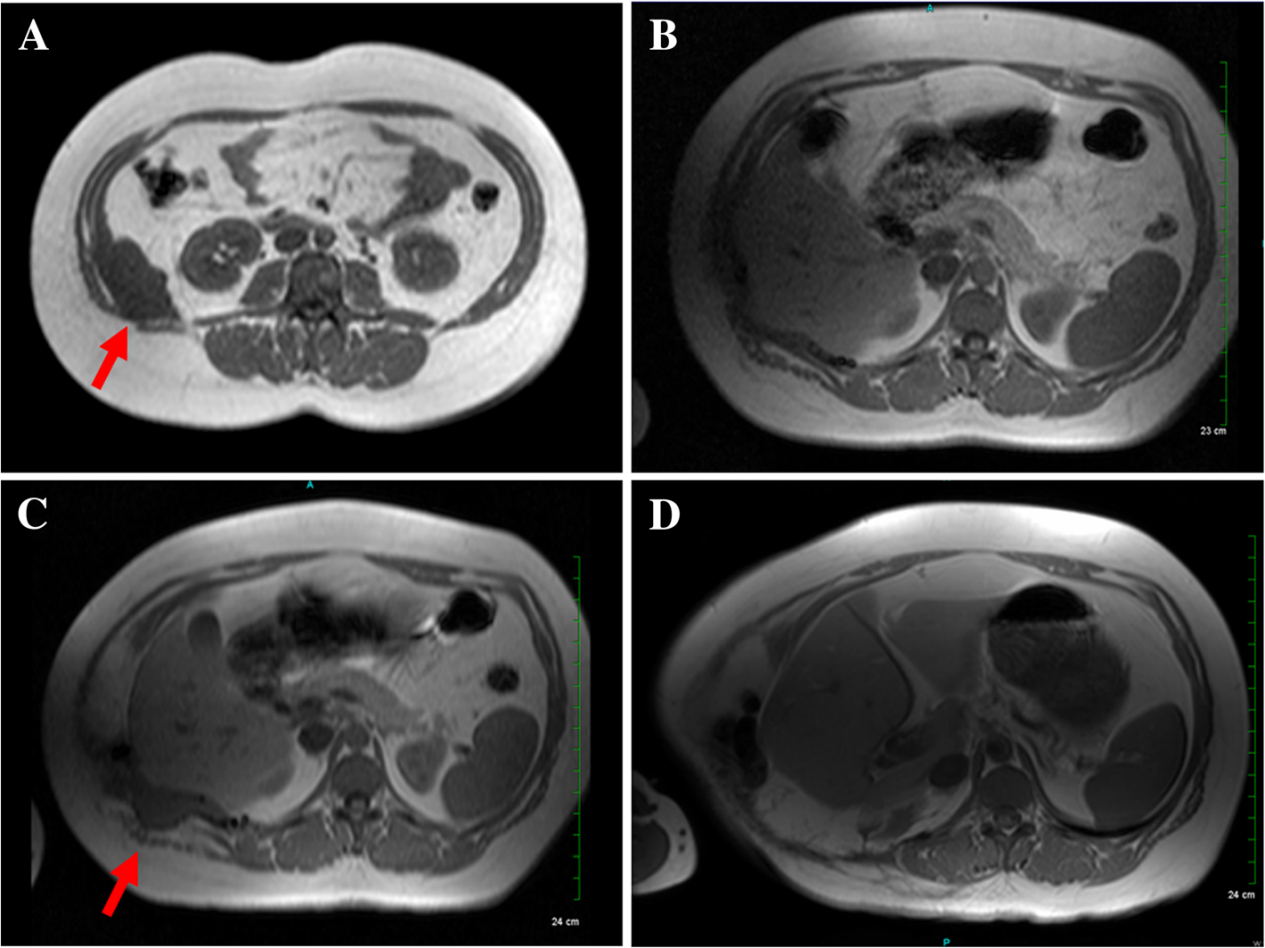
Serial magnetic resonance imaging (MRI) scans. (A) Taken at the initial diagnosis. The red arrow indicates 7.3 × 4-cm mass on the right posterior-lateral abdominal wall at the level of the flank and right kidney. (B) Taken after the initial surgery, showing no evidence of disease. (C) Taken 12 months after the initial surgery. The red arrow indicates 6.5 × 2.8-cm recurrent mass on the previous surgical bed. (D) Taken at 24 months after completing proton therapy. No evidence of re-recurrence of the tumor.
